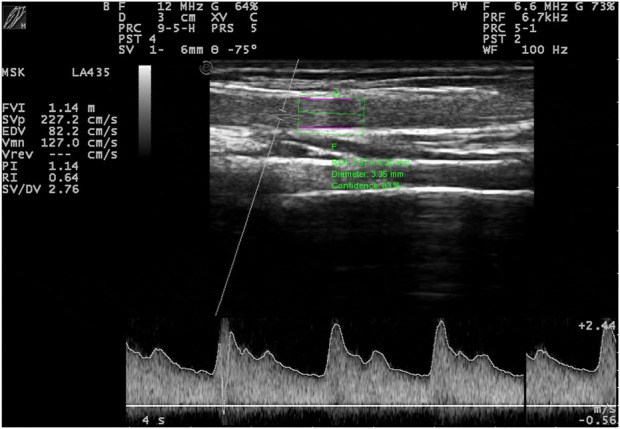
Ultrasound and doppler image of the brachial artery during visit two.
